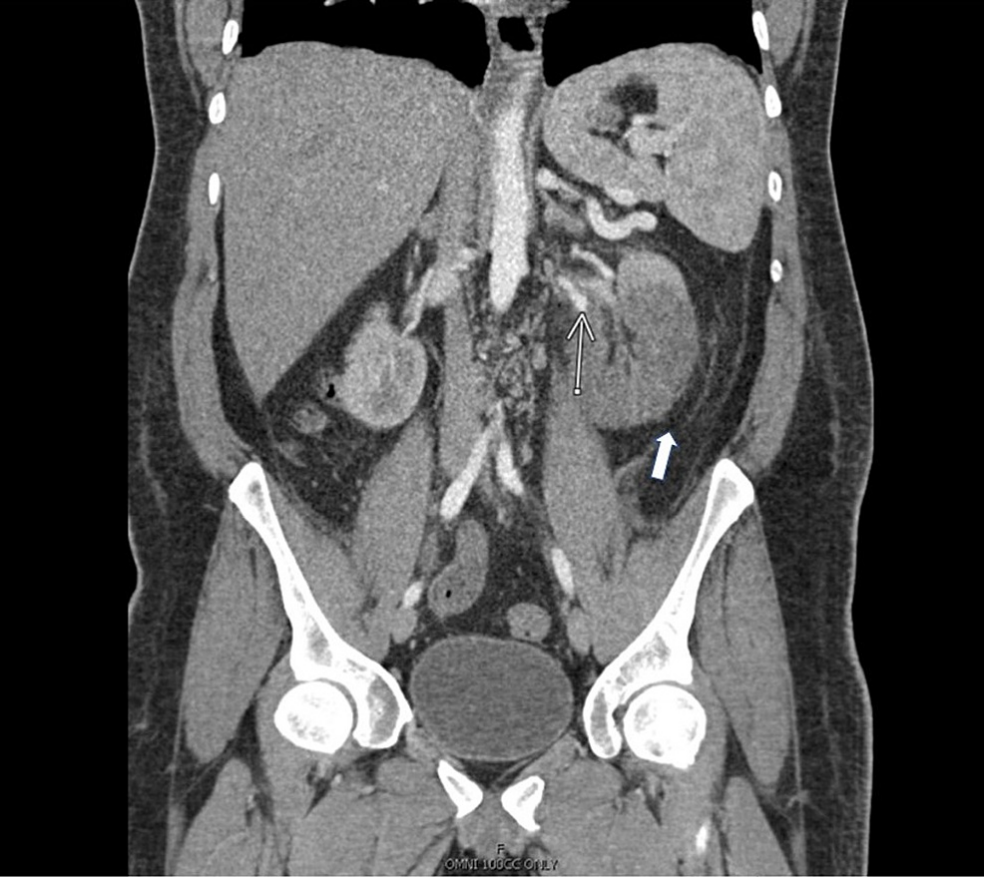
Computed tomography angiography of the abdomen. Note the reduced enhancement of the lower pole of the left kidney and a filling defect of the left renal artery (thin arrow). Also seen are perinephric fat stranding (thick arrow), patent left superior renal artery, and normal left upper renal pole enhancement.
